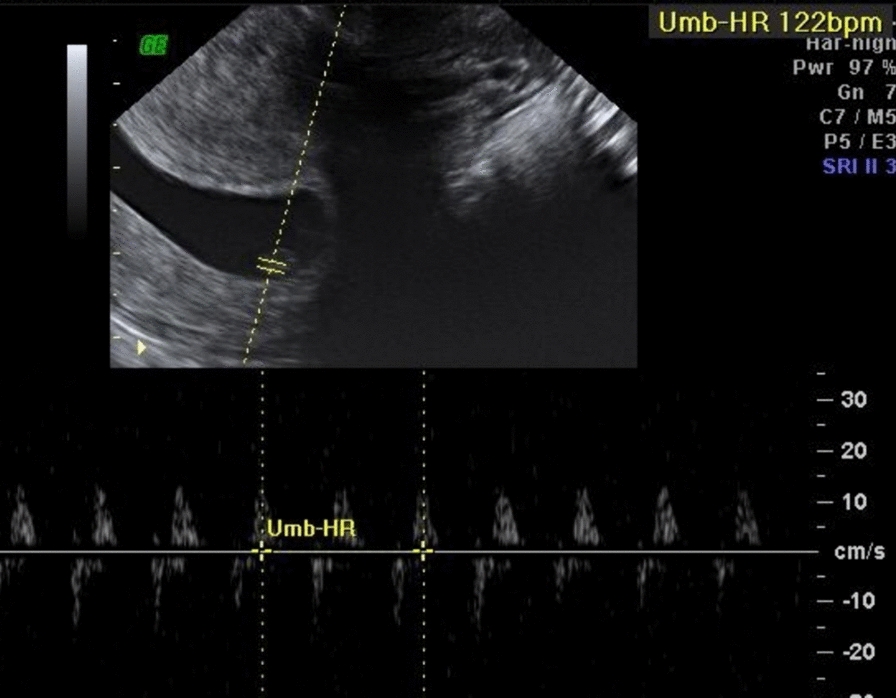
Ultrasound showing presence of fetal heartbeat in one uterus
